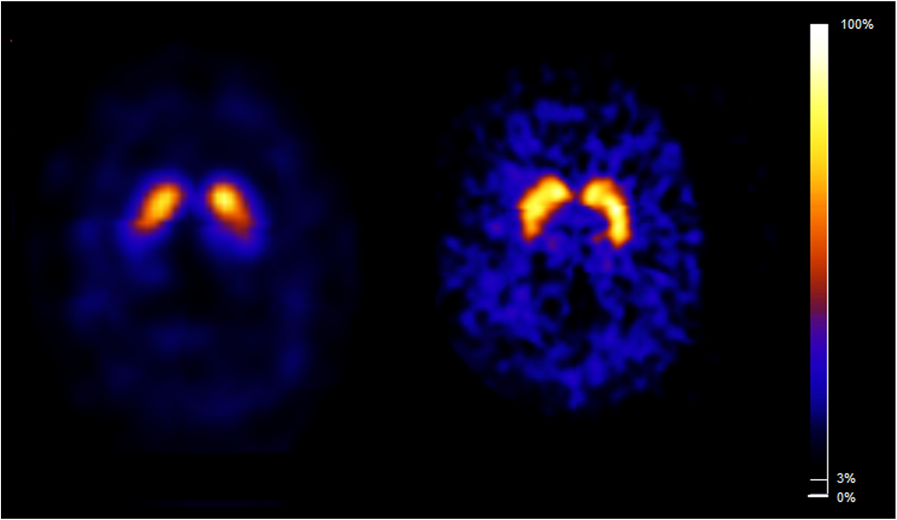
Discordant visual rating between both systems. Tranversal images of the [^123^I]FP-CIT SPECT scans rated visually as abnormal (PD-like; due to relatively low binding in the left putamen vs left caudate nucleus) on the NeuroFocus (left panel) and as “normal” on the InSPira (right panel) obtained in subject #8. Images are displayed in radiological orientation (left is right)
